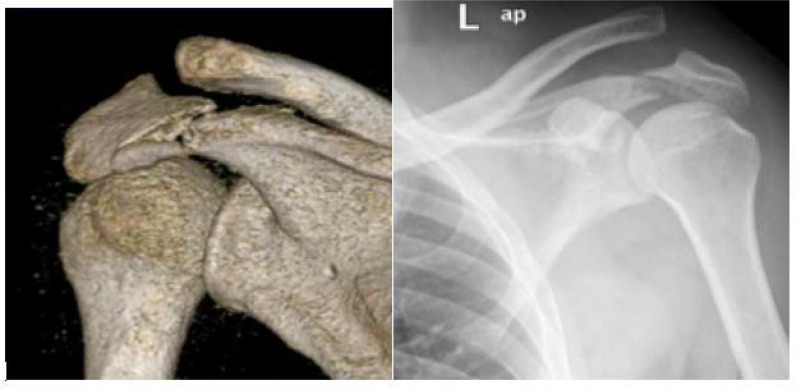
Three-dimensional reconstruction view and shoulder anteroposterior radiograph of a patient with left acromion-displaced fracture and acromioclavicular joint dislocation that was treated nonoperatively.
